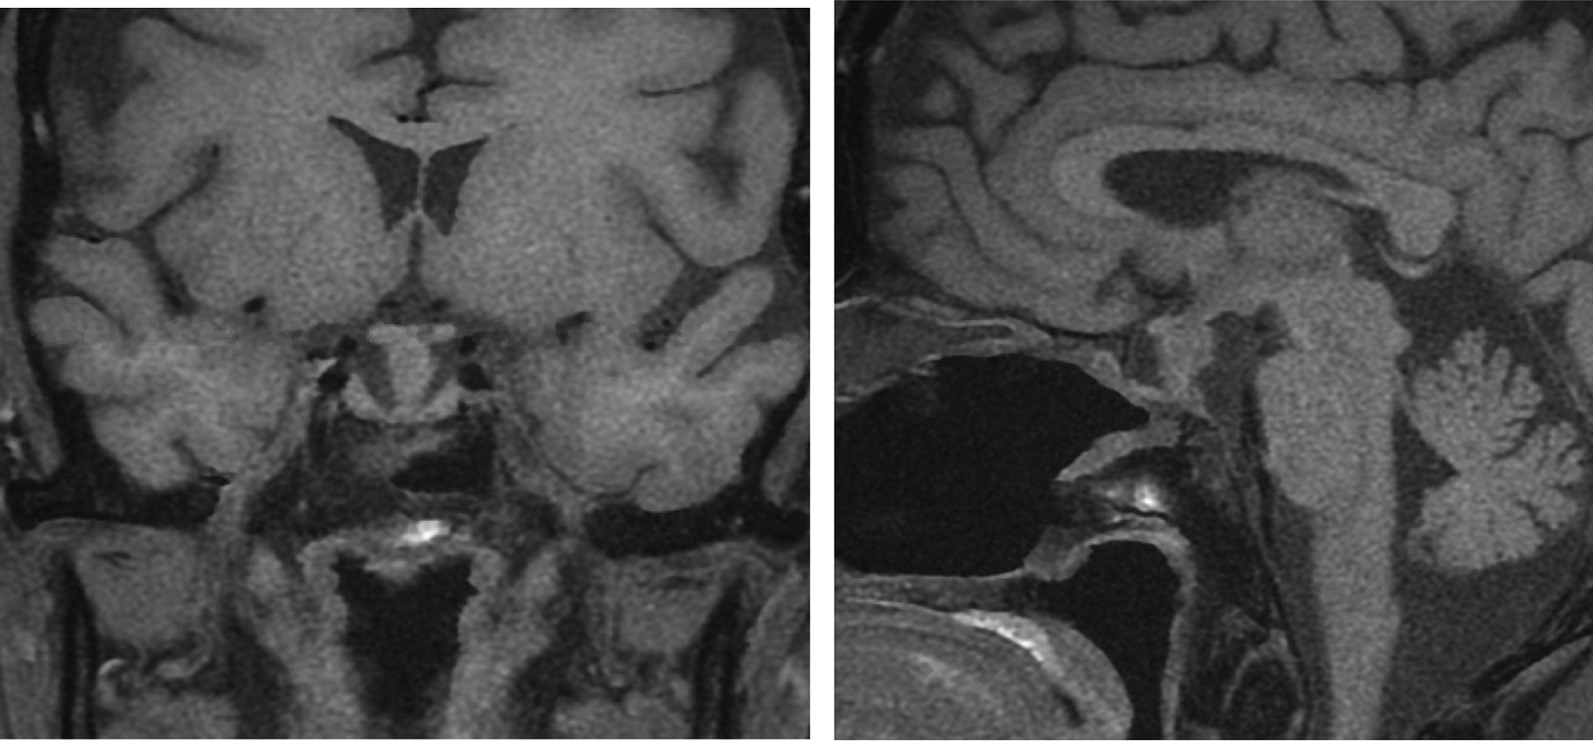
Last pituitary magnetic resonance imaging (MRI) 15/10/2019. Compared with the previous MRI dated on 15 April 2019, there is redemonstration of the residual enhancing lesion seen in the suprasellar region closely related to the pituitary stalk. Its residual remained stable in size; measures 12.5 × 11.5 mm in CC × AP dimensions in sagittal plane compared with 11.5 × 11.5 mm in previous study in same location. No other significant interval changes demonstrated
